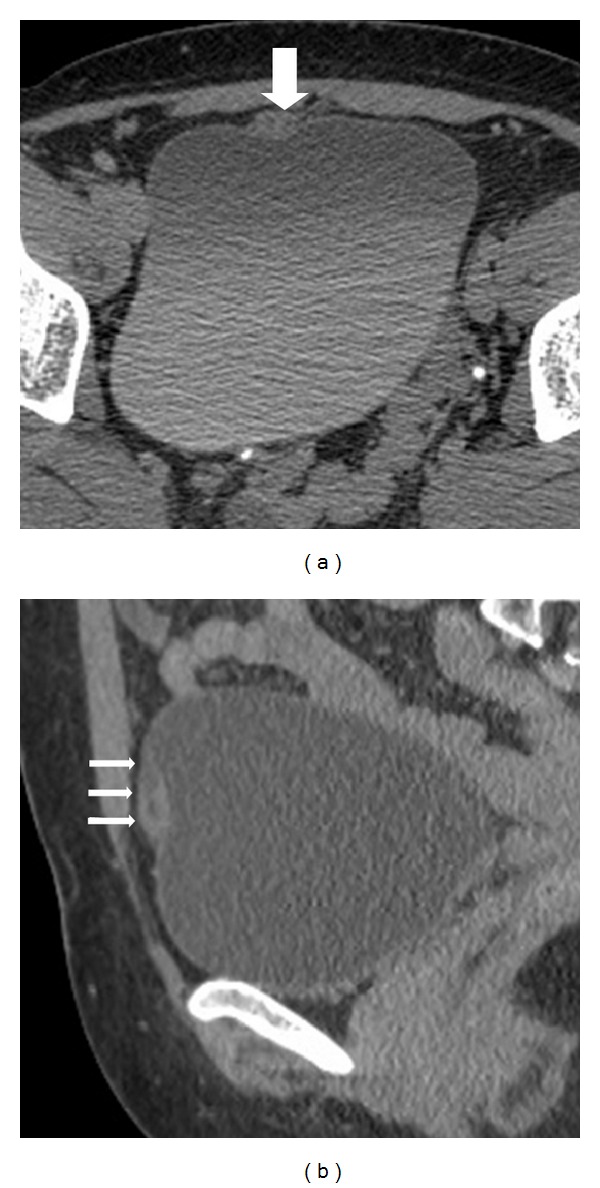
Contrast-enhanced CT revealed a fusiform mass with thick wall ((a), large white arrow on axial CT imaging) and internal millimetric cystic components overlying the anterosuperior portion of bladder ((b), small white arrows on sagittal CT imaging).
